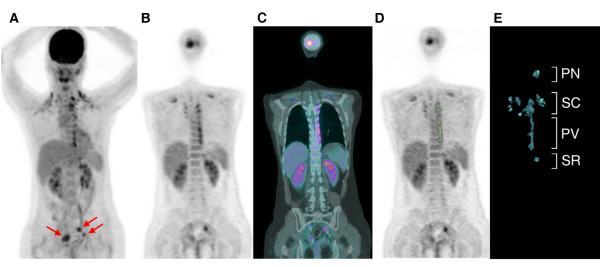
18F-FDG PET/CT scan of a 33-year-old woman with a history of cervical cancer treated with a radical hysterectomy: (A) maximum intensity projection, (B) coronal PET, and (C) coronal PET/CT fusion images showing activated BAT deposits in the posterior neck (PN), supraclavicular (SC), paravertebral (PV) and suprarenal (SR) areas. The hypermetabolic foci in the pelvis were locally recurrent tumors (red arrows) and the neoplastic status score was 3. (D) We used OsiriX 64-bit software with the seeded region growing method (green zone) to obtain the volume of interest (VOI) of activated BAT from the PET images. (E) Color image segmentation based on 3-dimensional clustering was used for display and volume computation. Total metabolic activity (TMA) was defined as the summation of the volume × standardized uptake value (SUV) within the VOIs of the activated BAT deposits.
